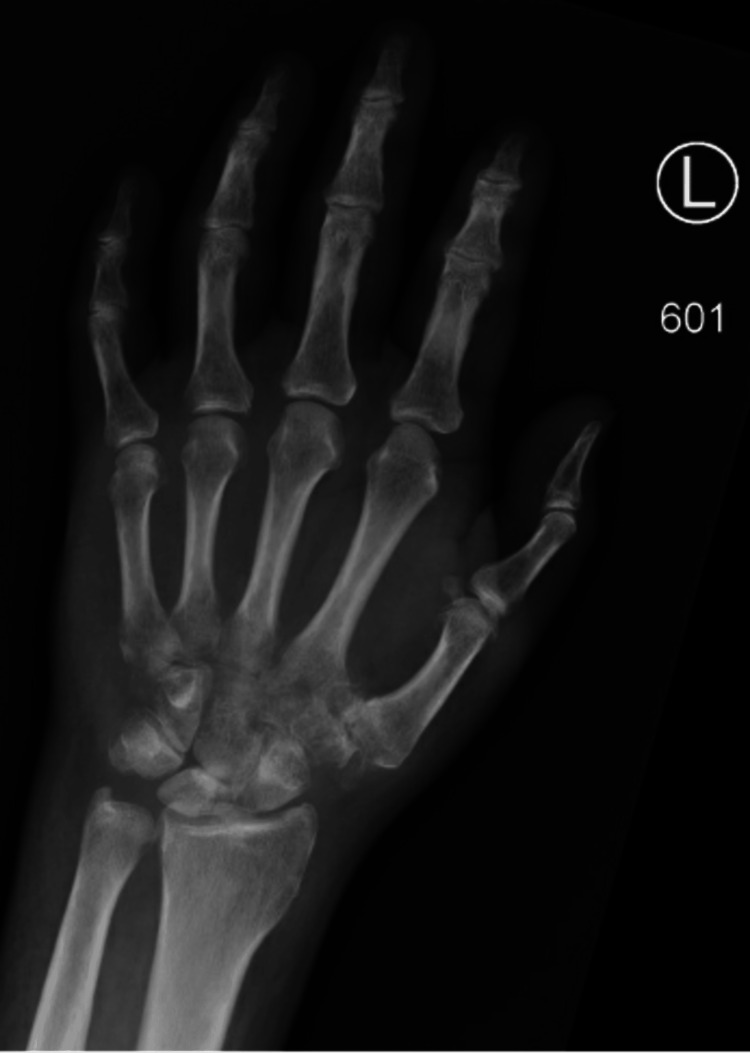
Follow-up left wrist radiograph. Radiograph, about six weeks after irrigation and debridement, showing extreme demineralization of most of the carpal bones and bases of the metacarpals, suggestive of osteomyelitis.
